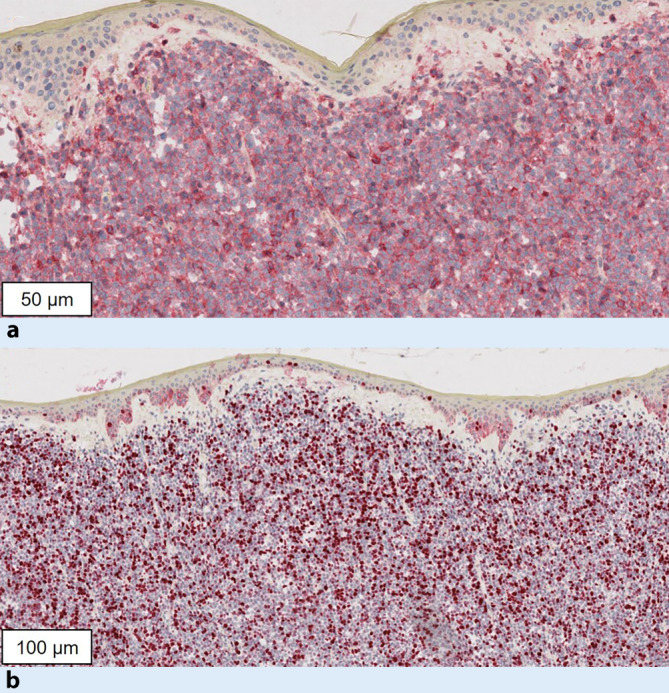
Eine Knochenmarkbiopsie, Abdomensonographie und Röntgenaufnahme des Thorax waren unauffällig.
